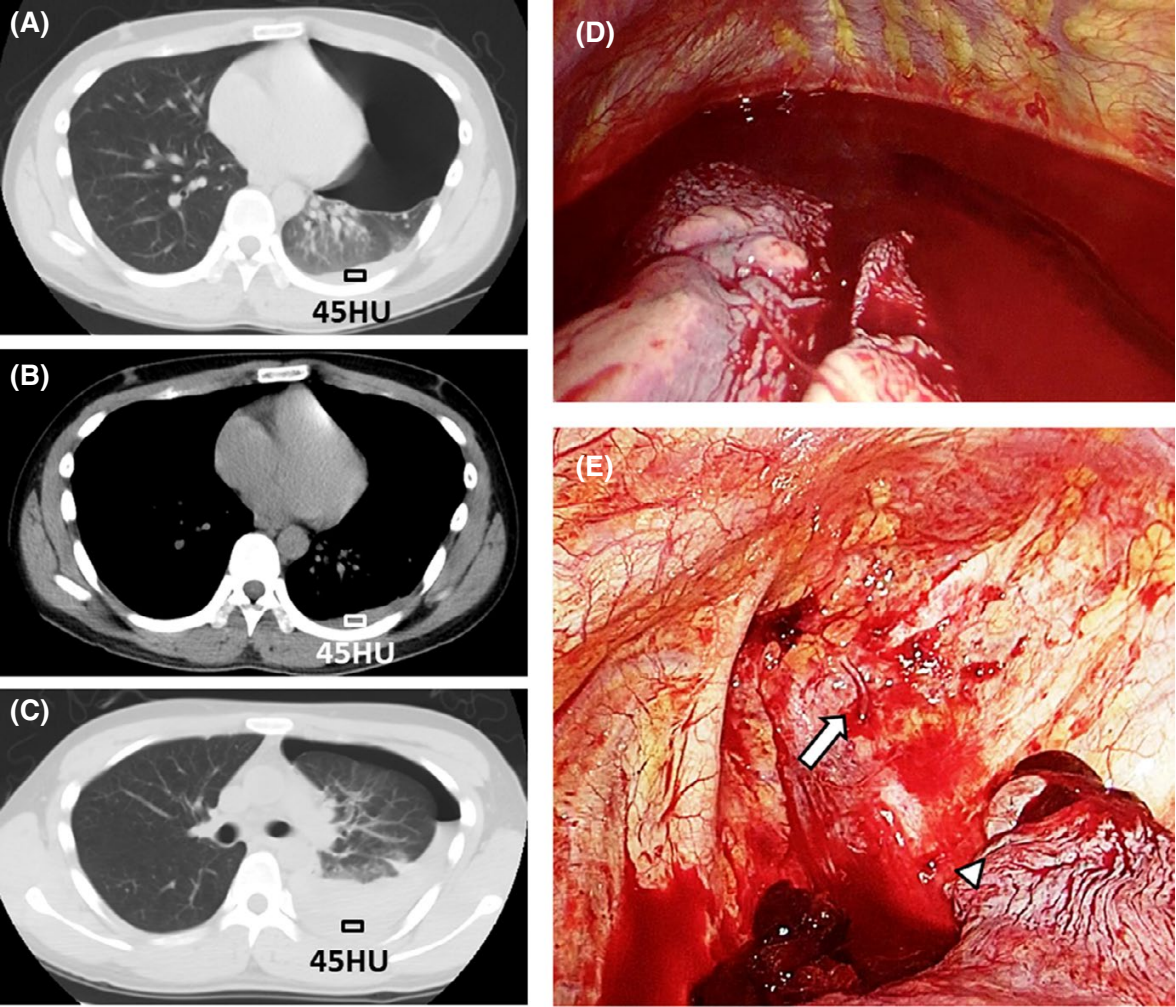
Chest CT on admission showing a tiny amount of pleural effusion with pneumothorax. The CT attenuation values of pleural effusion at this time were 45 Hounsfield Units, indicating that the pleural effusion was blood. (A: lung window, B: mediastinal window). Chest CT at the time of shock status showing rapid increase in pleural effusion. (C: lung window), (D) Intraoperative findings of video‐assisted thoracoscopic surgery showing accumulation of massive blood within the pleural space. (E) Intraoperative findings of video‐assisted thoracoscopic surgery showing that the formation of new blood vessels between the parietal and visceral pleura was the source of bleeding (arrow). The source of bleeding was located adjacent to ruptured bullae (triangle). CT, computed tomography; HU, Hounsefield Units
